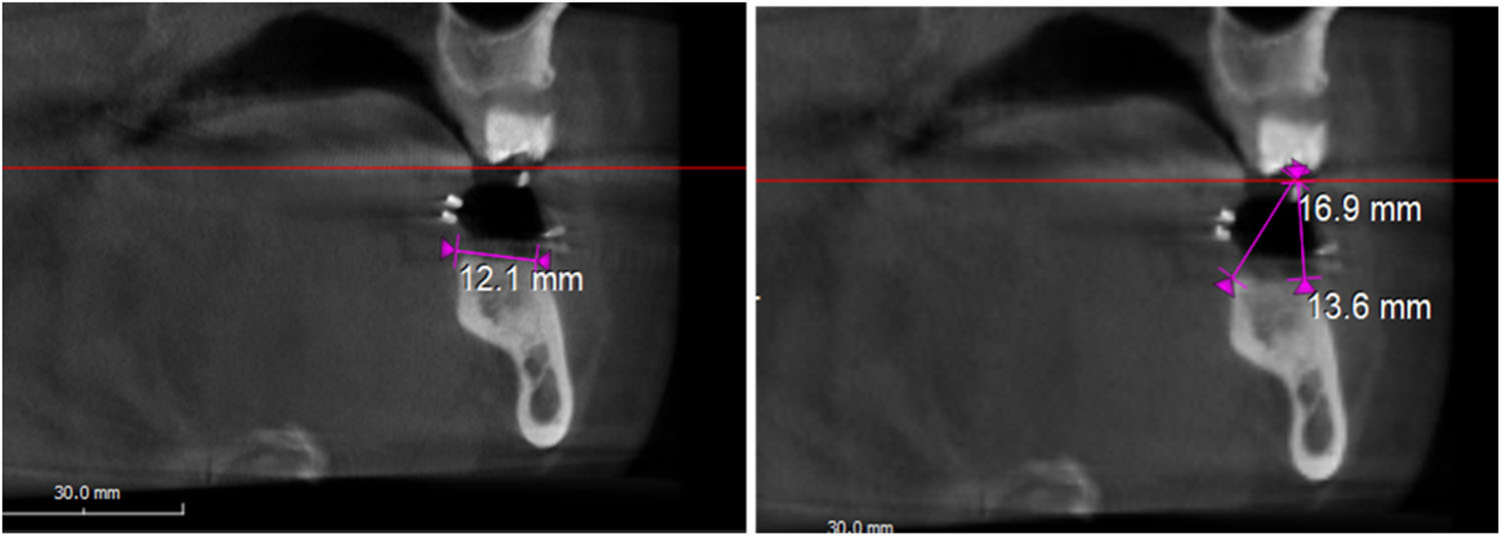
Radiographic measurements of the width of the alveolar ridge, the vertical distance (VR‐BC), and the vertical distance (VR‐LC).
